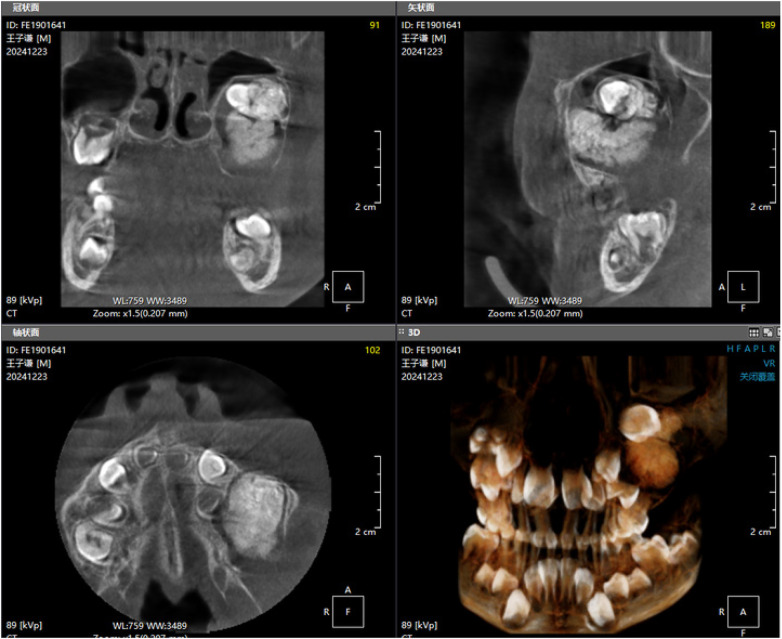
Preoperative CBCT image (2).
